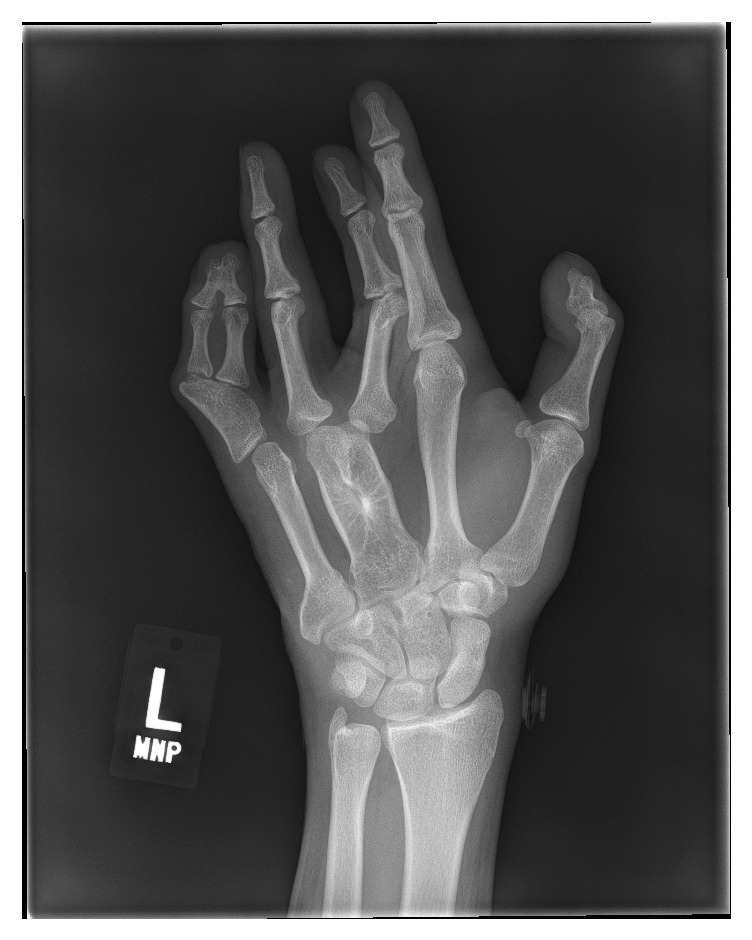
Hand X-ray exhibiting polysyndactyly of the 3rd and 4th metacarpals, and polydactyly of the middle and distal phalanges of the 5th finger. Subluxation of unknown age of the proximal phalanx of the 3rd finger was coincidentally noted.
